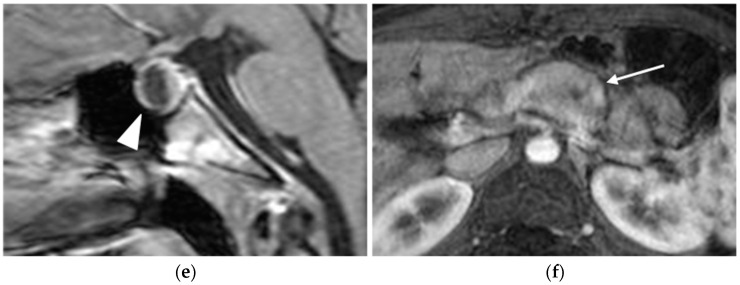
Young female MEN-1 patient, aged 19 at initial diagnosis. Ultrasound revealed a parathyroid adenoma (arrow in a,b) and MRI highlighted a pituitary macroadenoma (arrowhead in c–e) and a pancreatic NET (arrow in f).
